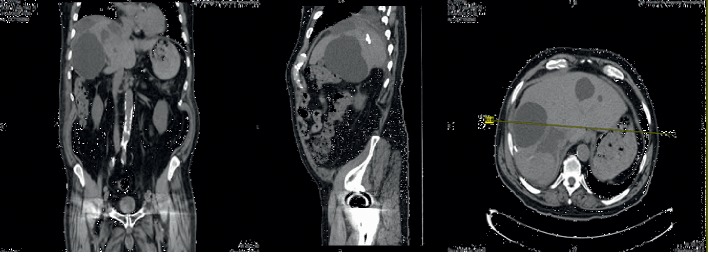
Postdrainage CT abdomen/pelvis showing significant reduction of the size of the cysts.
